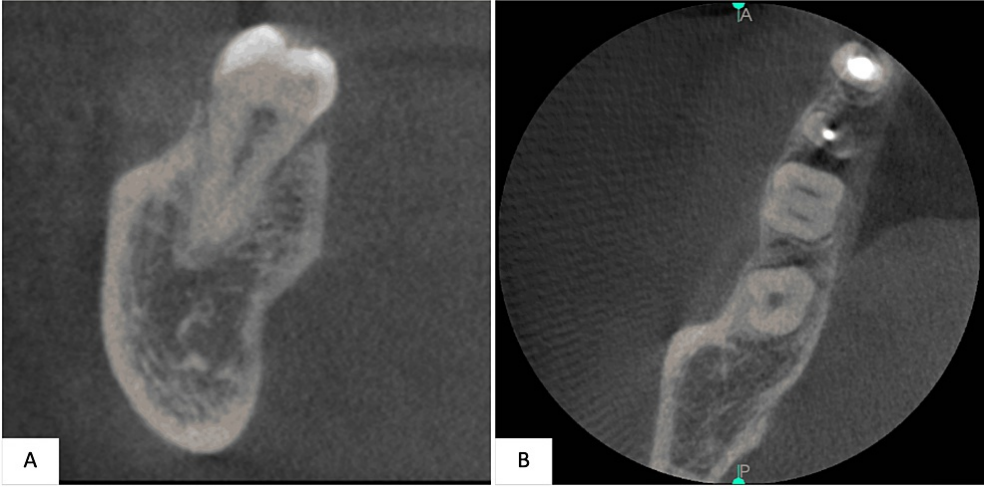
Cone-beam computed tomography (CBCT) for tooth #47 taken at 1mm slice thickness A: Coronal slicing B: Axial slicing
